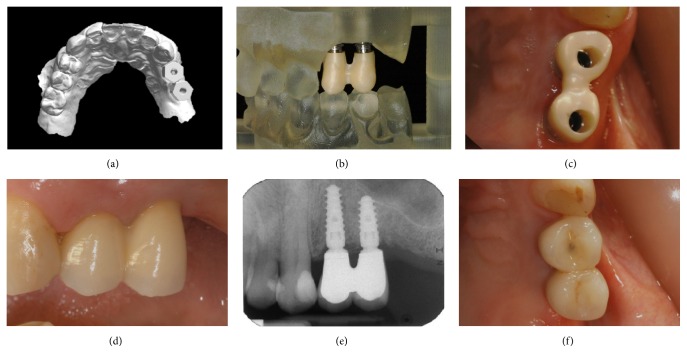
Three months after implant placement, the definitive intraoral impression is taken, and the definitive implant-supported restoration is fabricated and delivered to the patient: (a) digital impression with PEEK scanbodies; (b) the 3D-printed model with the zirconia framework; (c) the zirconia framework is seated on the definitive titanium abutments and checked for accuracy/precision (occlusal view); (d) delivery of the final zirconia-ceramic restoration, cemented on the final titanium abutments (side view); (e) periapical radiograph at the delivery of the final implant-supported restoration; (f) 6-month control of the final zirconia-ceramic restoration (occlusal view).
